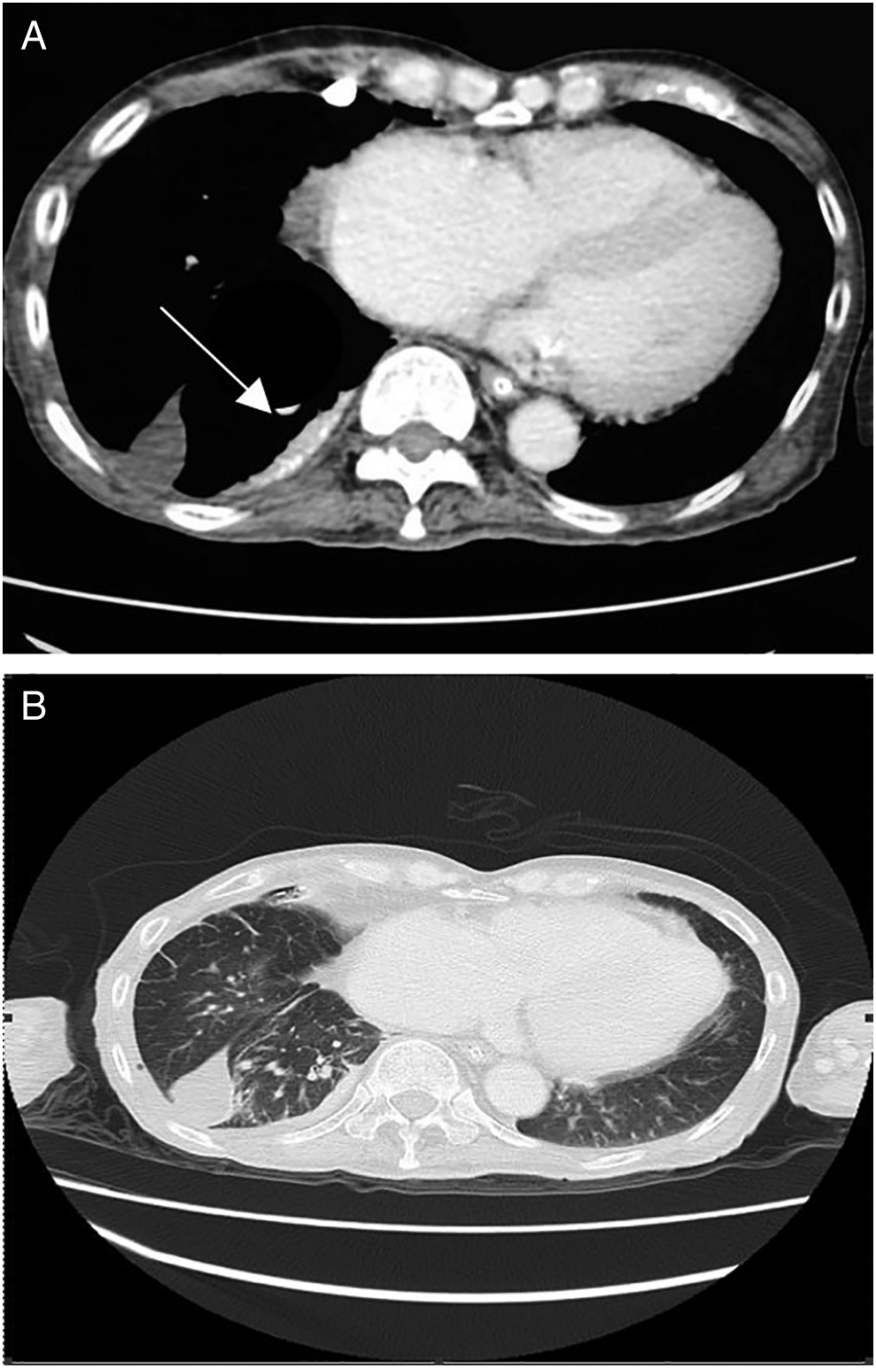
(A) Contrast‐enhanced computed tomography (CECT) thorax showed a 1.4 cm thick focal pleural plaque (white arrow) with calcification at the medio‐posterior aspect of right lower lobe. (B) Loculated fluid in the horizontal fissure and interlobular septal thickening.
